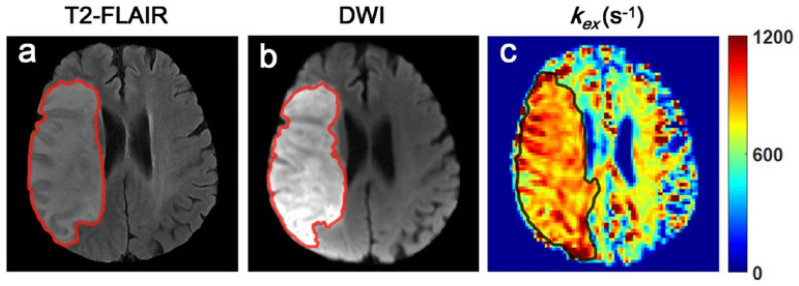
Different MRI images from a representative ischemic stroke patient showing the infarct lesion area. (a) T_2_-weighted fluid-attenuated inversion recovery (T_2_-FLAIR) image. (b) DWI. (c) *k_ex_* MRI. The *k_ex_* map reflects a larger extent of the infarct lesion with an elevated signal. (Reprinted by permission from J Neurosci Methods. 2020; (346), 108926. Figure 4).
